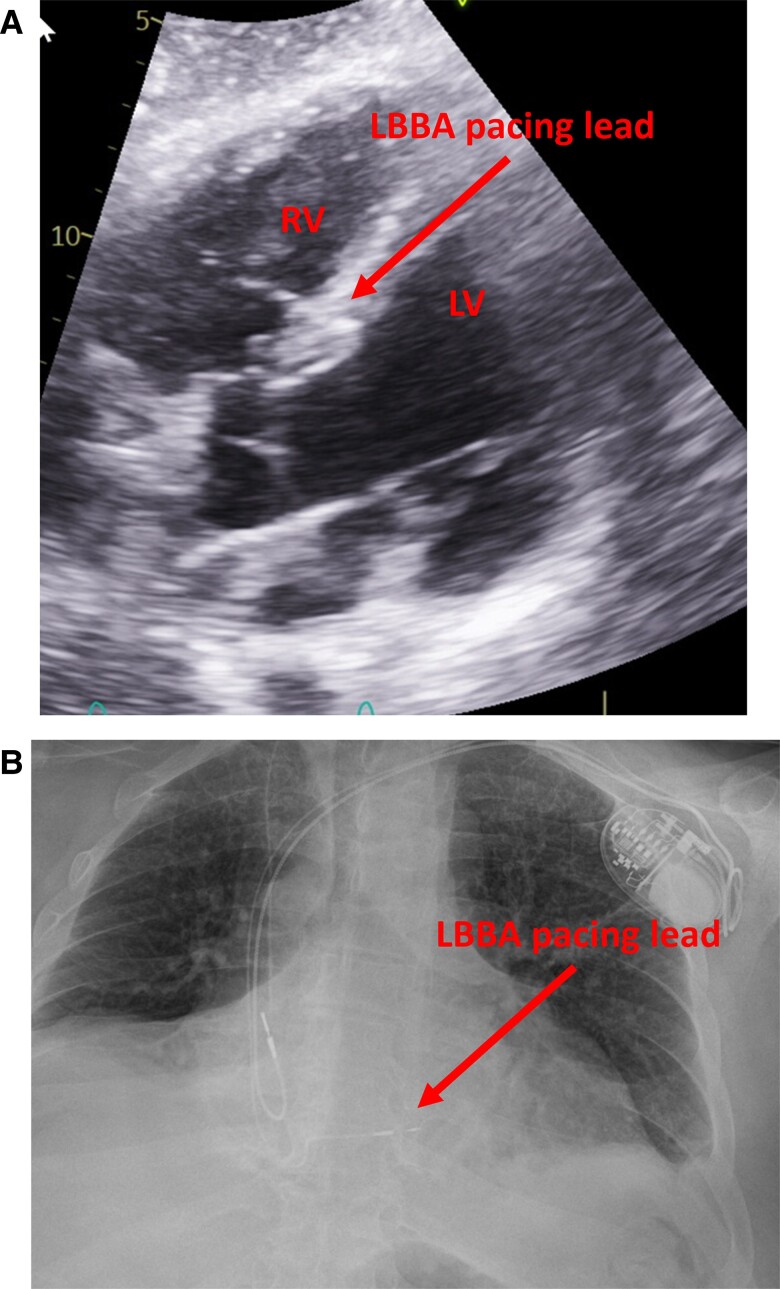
Post-op imaging demonstrating expected lead position. (*A*) ECHO imaging showing the ventricular lead deeply embedded in the basal ventricular septum with the tip abutting the LV endocardium. (*B*) Post-operative X-ray showing the septal location of the RV lead.
